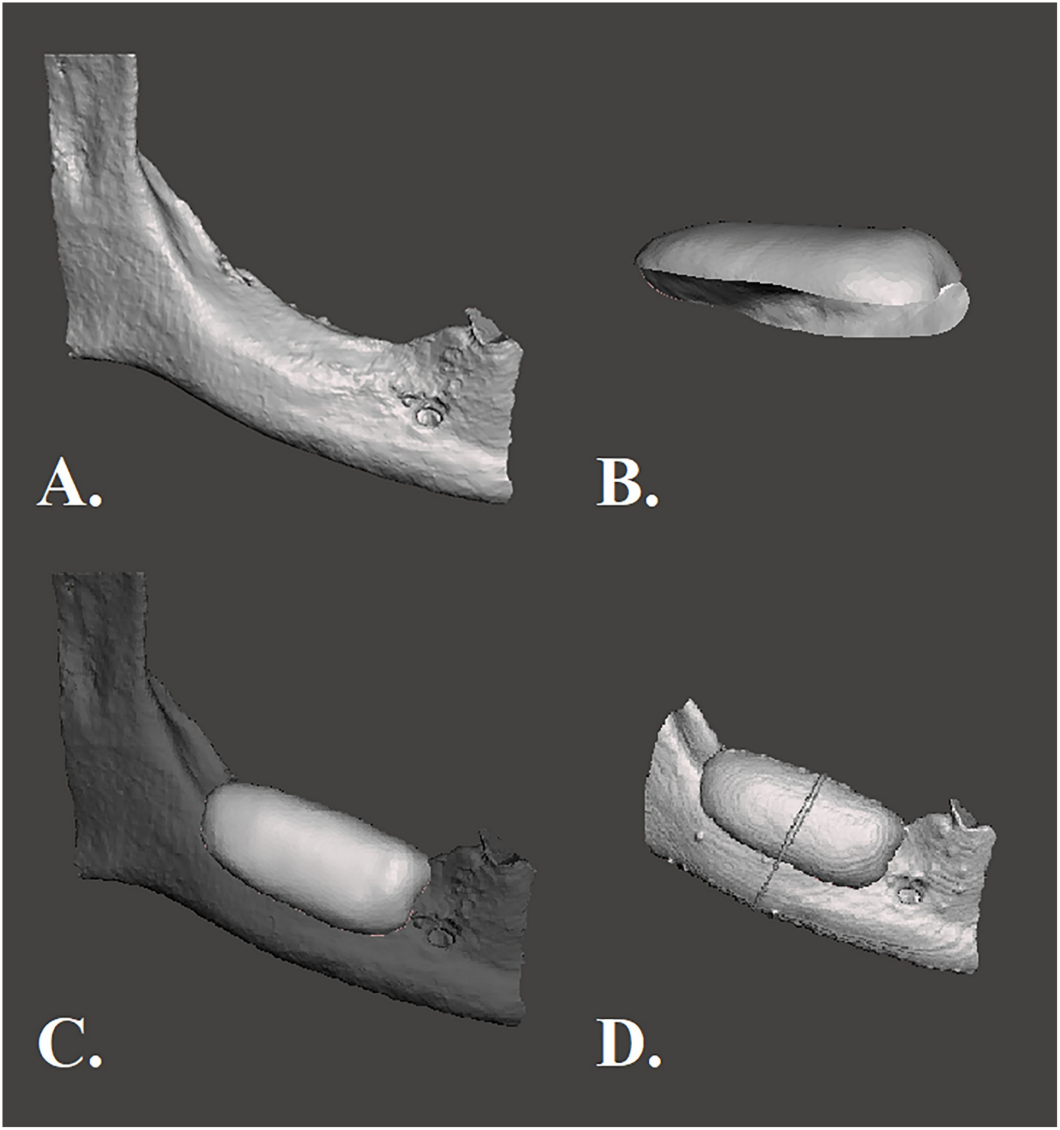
Representative 3D models of an atrophic mandible combined with one out of three large grafts. (A) Frontal view of a CAD mandibular model. (B) Lateroinferior view of a CAD large mandibular graft. (C) Frontal view of the graft fitted on the recipient site of the mandible. (D) Frontal CBCT view of the fitted graft.
